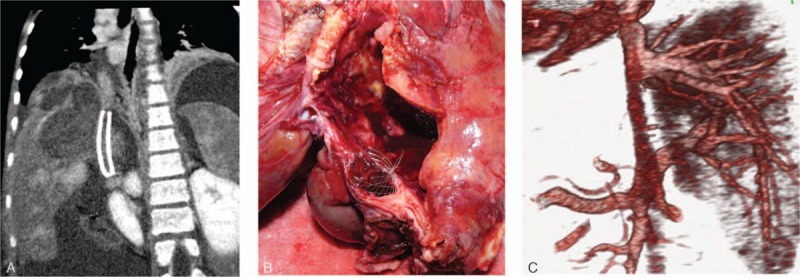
Coronal CT image obtained after placement of a stent to the VCI by an interventional radiological method in order to relieve BCS (A); severe venous injury secondary to stenting is shown in the explanted retrohepatic VCI of the liver (B). CT image showing venous drainage after the LDLT (C). BCS = Budd–Chiari Syndrome, CT = computed tomography, LDLT = living donor liver transplantation, VCI = vena cava inferior.
